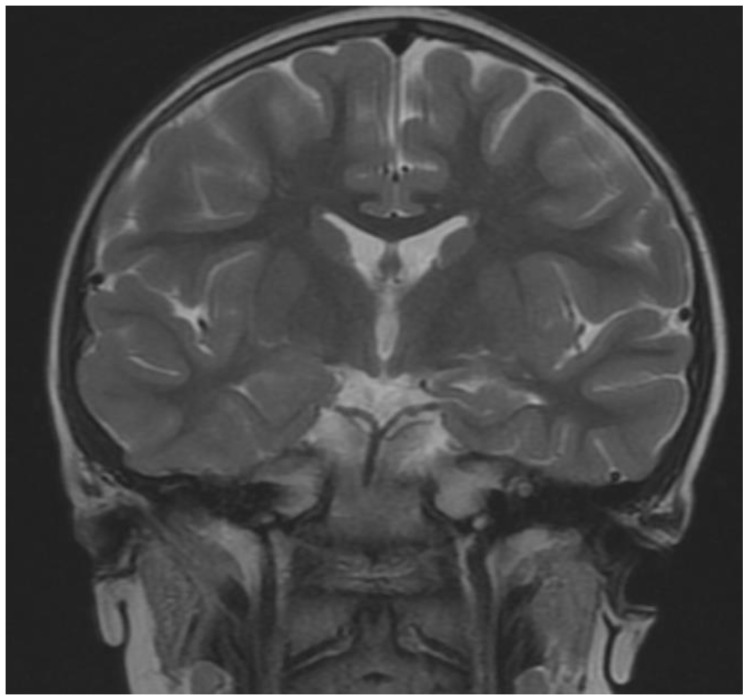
Coronal B (T2-weighted sequence)—Thicker cortical layer on the right side.
